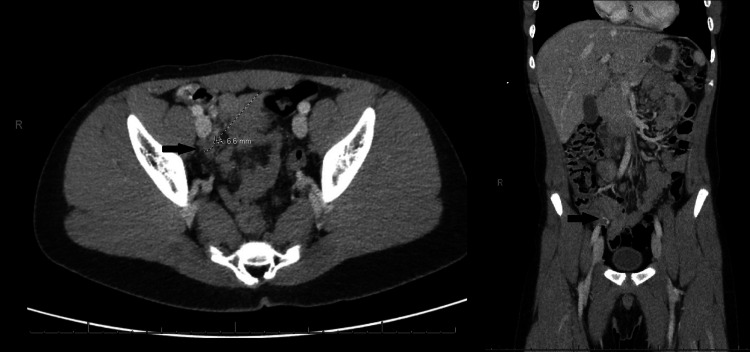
CT showing a 7-mm fluid-filled appendix with a “mildly enhancing” wall and small free fluid.
